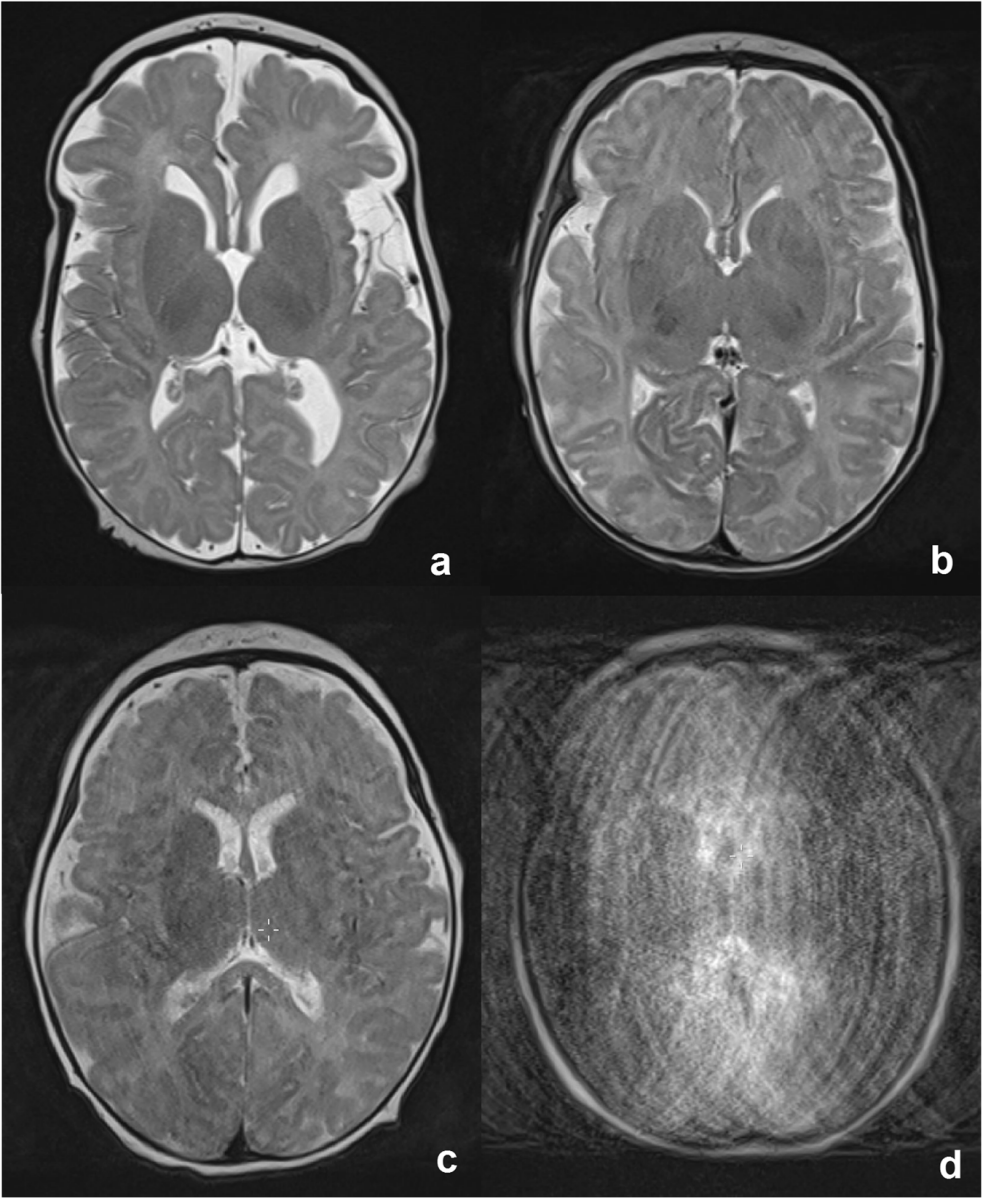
Examples of the different quality scores based on a T2-weighted turbo spin echo sequence of the brain at the level of the basal ganglia. a Score of 4 with excellent image quality. b Score of 3 with slight motion artefacts, primarily affecting the frontal lobe. c Score of 2 with marked artefacts. Anatomic landmarks are still visible, but details, such as cortico-medullary differentiation, are only partially assessable. d Nondiagnostic image with severe artefacts, corresponding to a score of 1
